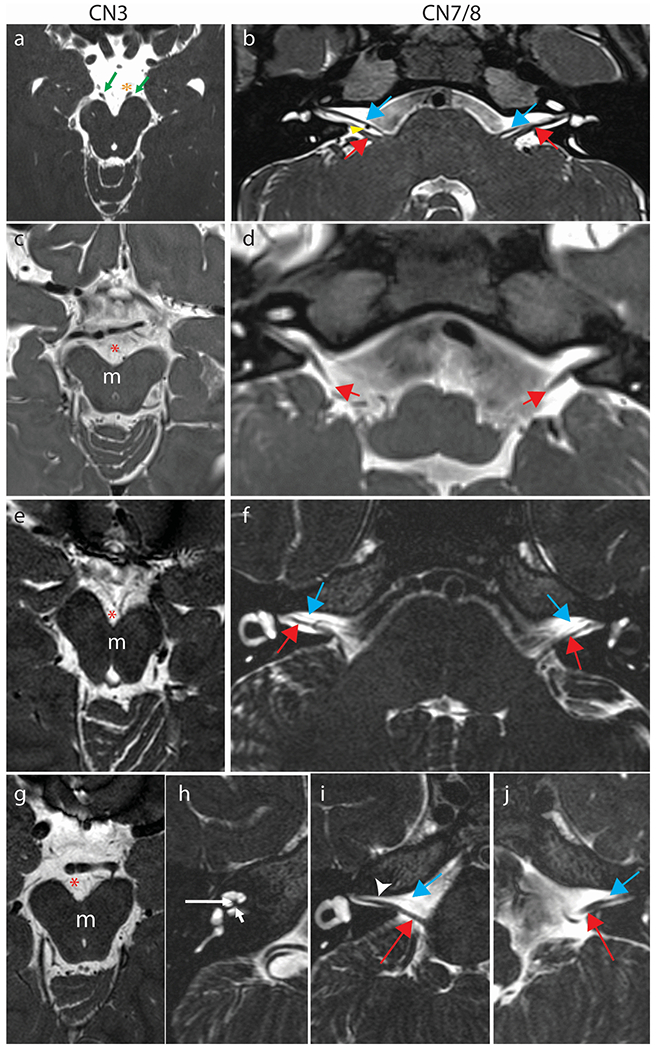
Cranial nerve MR imaging. Oculomotor nerve (a,c,e,g) and facial nerve (b,d,f,i,j) and cochlear nerve (h) imaging in comparison subjects (a,b), Subjects IV (c,d), X (e,f), and VII (g-j). (a) Normal CN III. 3 Tesla Axial constructive interference in steady-state (CISS) image through the midbrain at the level of the interpeduncular cistern showing normal CNs III emerging from the ventral aspect of the cerebral peduncles (green arrows). The oculomotor nerves course inferolaterally to the cavernous sinuses in close proximity to the posterior cerebral artery (PCA) on each side (orange asterisk left PCA). (b) Normal CN VII and VIII. 3 Tesla Axial sampling perfection with application optimized contrasts using different flip angle evolution (SPACE) image demonstrating normal anatomy of CN VII (blue arrows) coursing parallel and ventral to CN VIII (red arrows) on each side. The anterior inferior cerebellar artery flow void is seen between the cranial nerves on the right (yellow arrowhead). (c,e,g) Axial sampling perfection with application optimized contrasts using different flip angle evolution (SPACE) images (c) or Axial T2 weighted (e,g) images at the level of the interpeduncular cistern (red asterisk) show apparent absence of the cisternal segments of cranial nerves III which usually course anterolaterally from the ventral aspect of the midbrain (m) on each side. (d,f,i,j) At the level of the superior internal auditory meati, the cisternal segments of cranial nerve VIII are well seen bilaterally (red arrows, d [SPACE], f, i, j [axial T2 weighted]). Cranial nerves VII, which ordinarily course anterior and parallel to VIII are either not seen on either side (d) or appear markedly hypoplastic (blue arrows in f, i, j) and are faintly visualized coursing ventral and parallel to the cranial nerves VIII (red arrows). The right internal auditory meatus is asymmetrically narrowed (i, arrowhead). (h) In subject VII, who has right-sided hearing loss, the cochlear modiolus is thickened and misshapen (longer white arrow) with severe stenosis/atresia of the cochlear aperture (short white arrow). The cochlear division of the right CN VIII, which usually courses through the cochlear aperture, is not visible.
